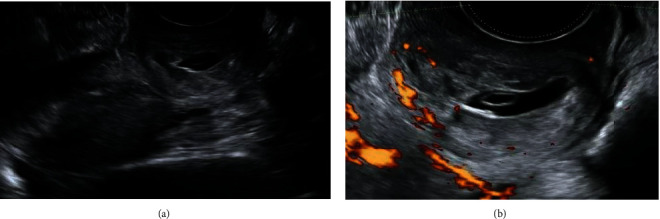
Cervical pregnancy at presentation (a) 2D transvaginal sonography in sagittal view shows an empty uterine cavity and closed internal os with ballooned cervical canal containing gestational tissue with (b) evidence of peritrophoblastic vascularity.
